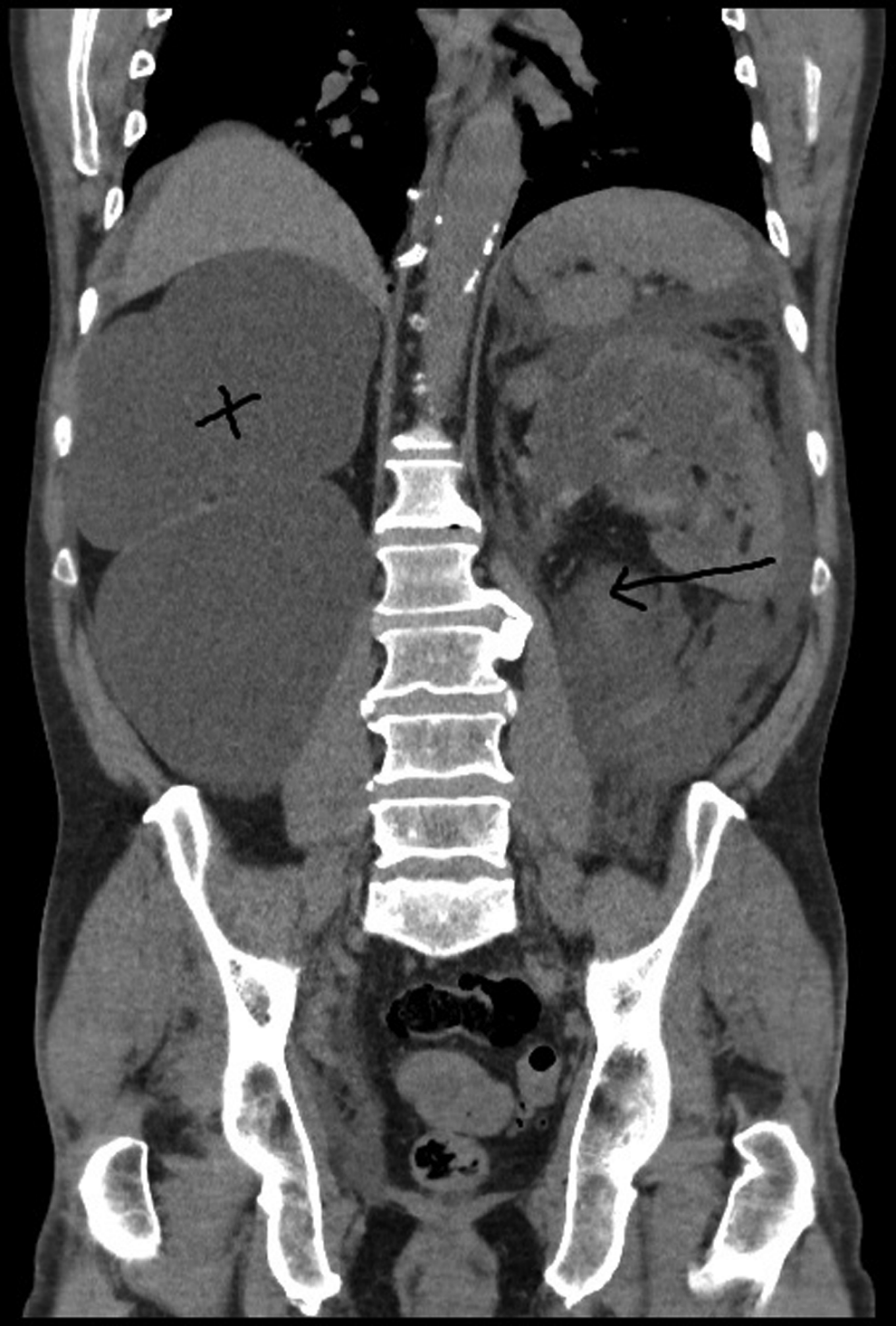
Coronal section of CT showing right nonfunctioning kidney (*cross*) with left retroperitoneal collection (*arrow*).
